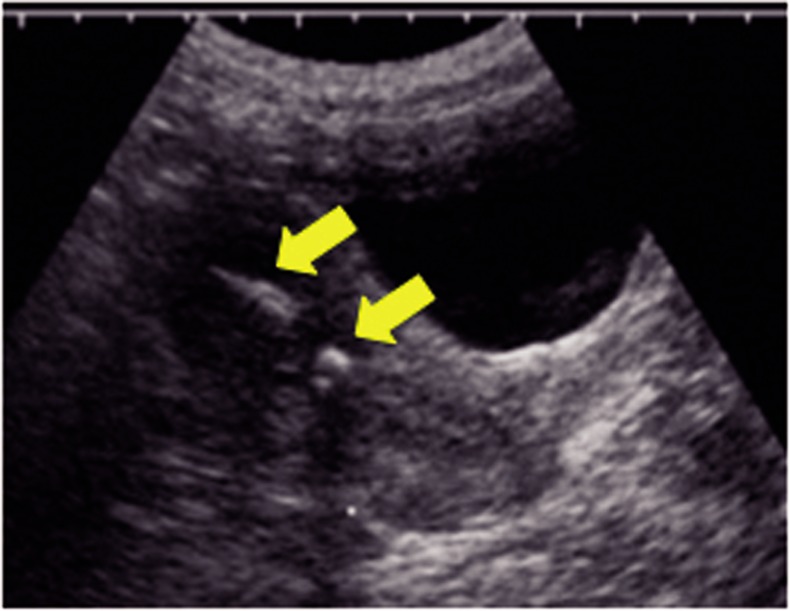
Bone fragments within the endometrium visualized sonographically. Arrows point to bone fragments.
